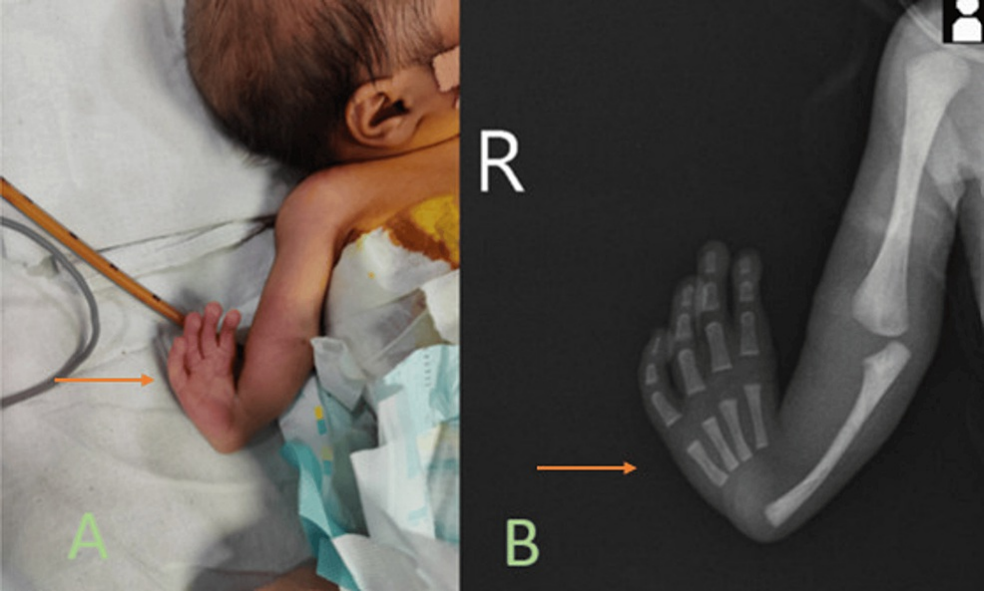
Clicked Image (A) and Antero-posterior X-ray of right arm showing complete absence of the radius and thumb of the right hand suggestive of radial ray type 4 with radial deviation of the fingers (orange arrows).
